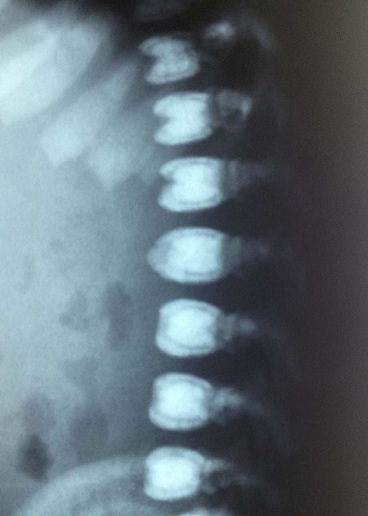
Lateral spine radiograph. Note vertebral sclerosis resulting in “sandwich vertebrae appearance’’
